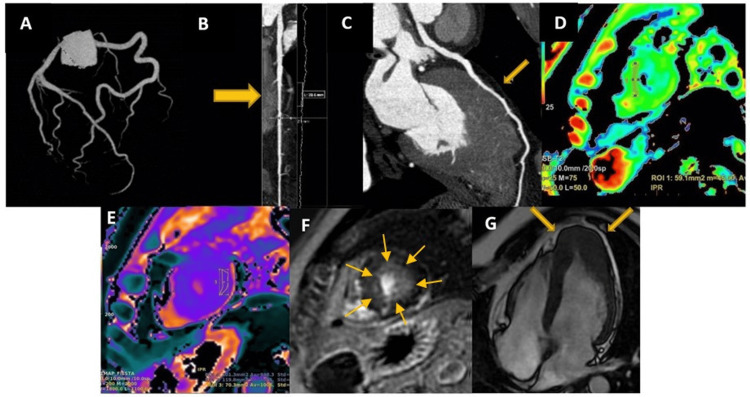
CCTA and CMR CCTA: coronary computed tomography angiography; ECV: external electrical cardioversion; LGE: late gadolinium enhancement; SSFP: steady-state free precession Image A-C: CCTA showing no obstructive coronary disease; a superficial myocardial bridge is seen in the mid-left anterior descending artery (arrow).  Image D: T2 mapping demonstrating increased values in the apical segment (56 ms). Image E: Native T1 mapping elevated (1006-1013 ms), ECV of 30%. Image F: LGE with subendocardial enhancement at the apex (arrow). Image G: Cine SSFP four-chamber view showing apical hypertrophy with a “spade-like” configuration
